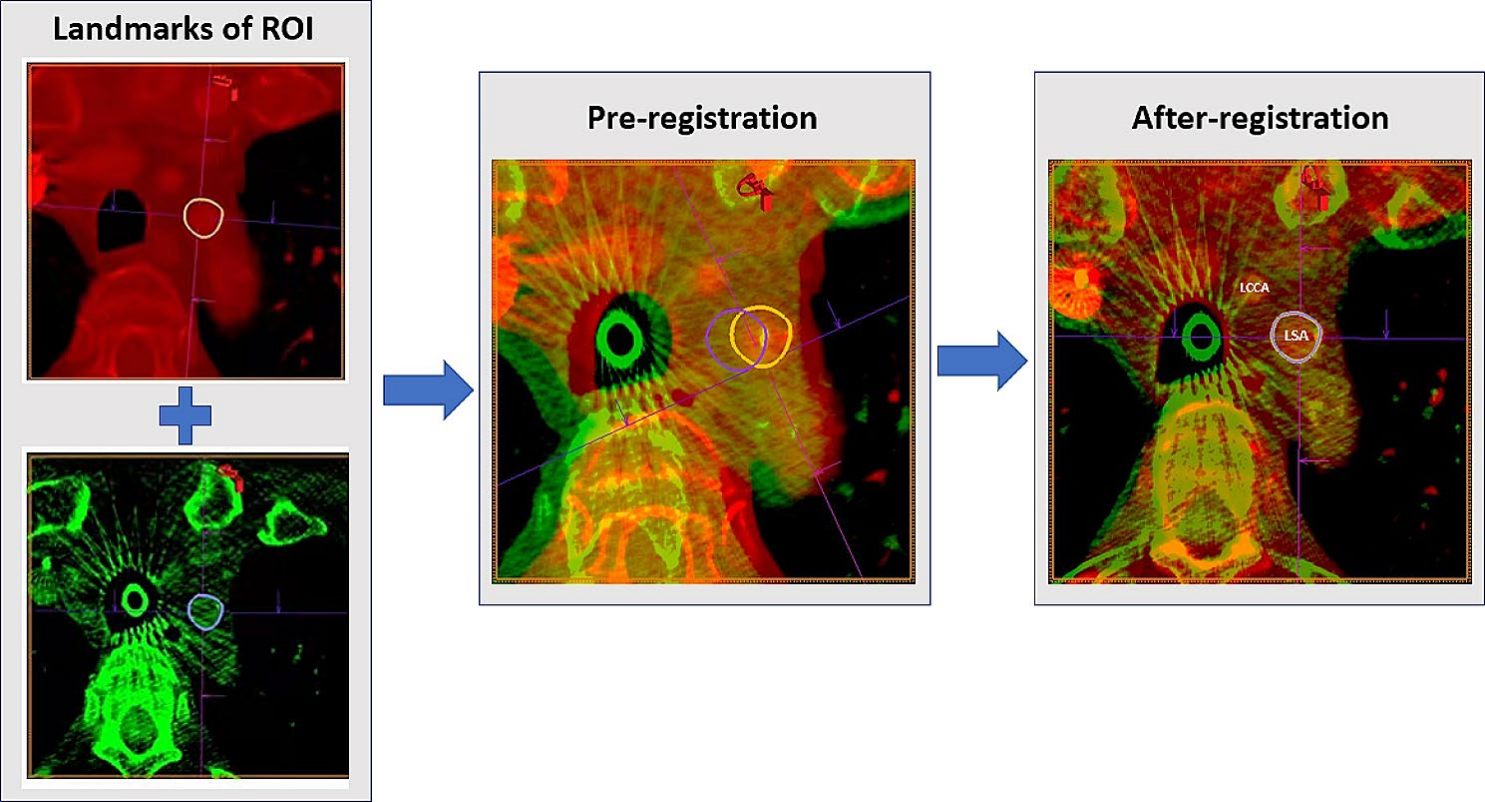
Image registration of preoperational three-dimensional CTA (red modality, upper left) and intraoperative CBCT (green modality, bottom left). First, the LSA ostium (the ROI in this study) was marked in both CTA (yellow circle) and CBCT (purple circle) modalities. Second, automatic registration, which was based on bony landmarks by default, could be performed to ensure the two modalities are in the same field of view. Thirdly, landmarks of the LSA were aligned from coronal view and checked from other planers to complete the registration
